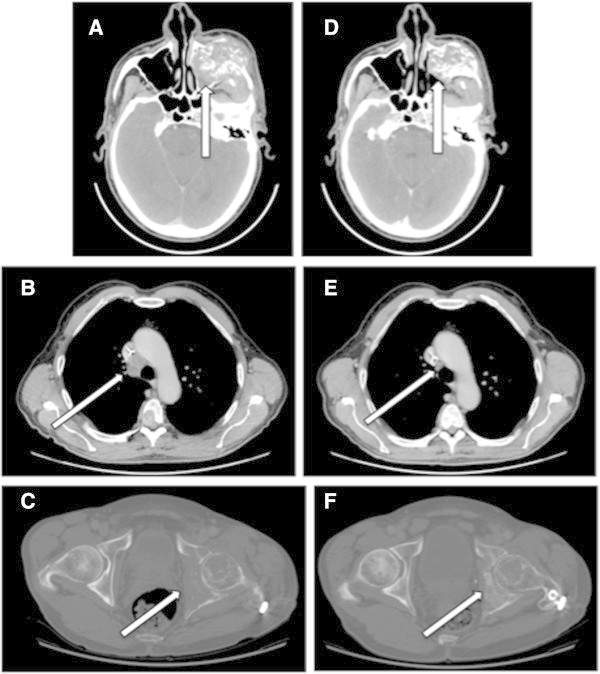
Comparison of CT scans realized before and after two months of treatment with a BRAF inhibitor. A, B, C: CT realized on April 2012. D, E, F: CT realized on June 2012 A, D: Reduction of tissue infiltration around malar bone destruction, especially on the sinusal component. B, E (after injection of contrast agent): Complete regression of mediastinal lymph nodes in station 4R. C, F (bone window): Left iliac bone regeneration. Notice cortical bone regeneration of arcuate line of ilium.
